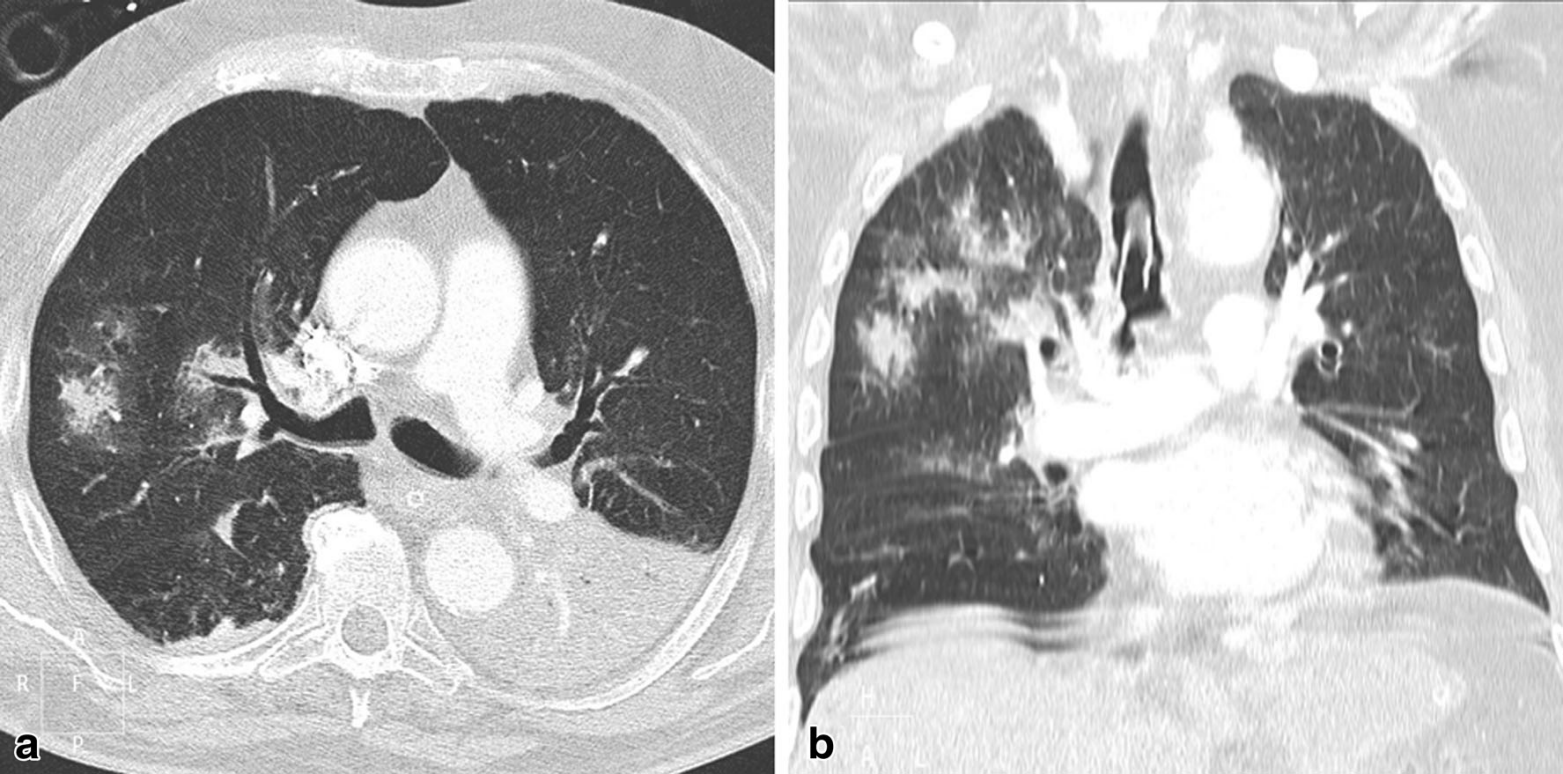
A 67-year-old female patient presented with new onset of chest pain and dyspnoea. The patient was diagnosed with an intertrochanteric femur fracture the day before and initial thoracic CT showed no abnormalities. Pulmonary CTA was ordered in order to rule out pulmonary embolism. (a-b) Thoracic CTA, (a) axial and (b) coronal, showed alveolar- and ground glass opacities, and nodules with halo sign distributed predominantly in the right upper lobe, and a left lower lobe consolidation. The nodular and ground glass pattern in the clinical context raised the diagnosis of pulmonary fat embolism
